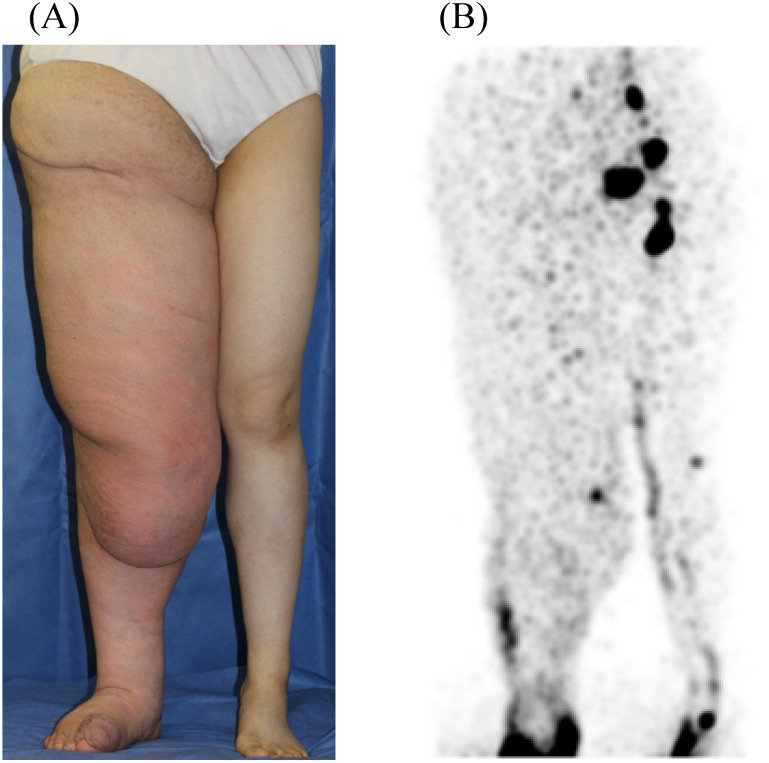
Fig. 1 (A) Frontal view of the lower limbs. (B) Radionuclide lymphoscintigraphy. Increased radiotracer accumulation in the right calf and no lymph nodes at the right groin.
